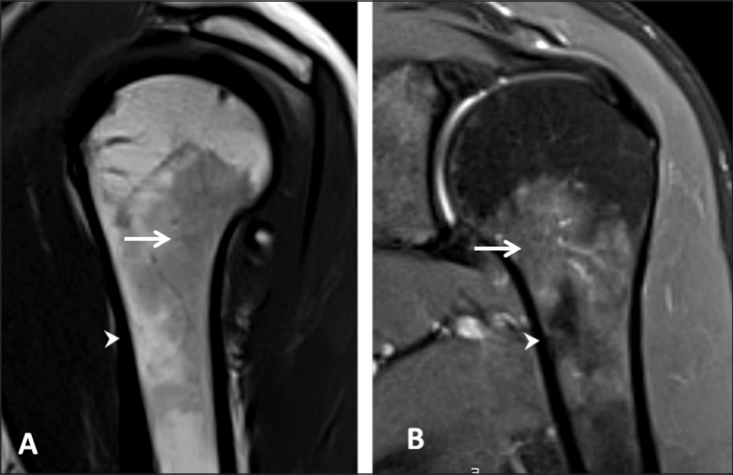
Normal red bone marrow in a young adult. (A) Sagittal oblique T1-weighted and (B) Coronal oblique fat-suppressed PD-weighted MR images detect areas of red marrow in the proximal humeral diaphysis with low signal intensity on T1 (arrow, A) and increased signal on fat-suppressed PD (arrow, B). This is usually observed bilaterally and without epiphyseal involvement. Cortical bone has a low signal intensity on both sequences (arrowhead, A and B).
